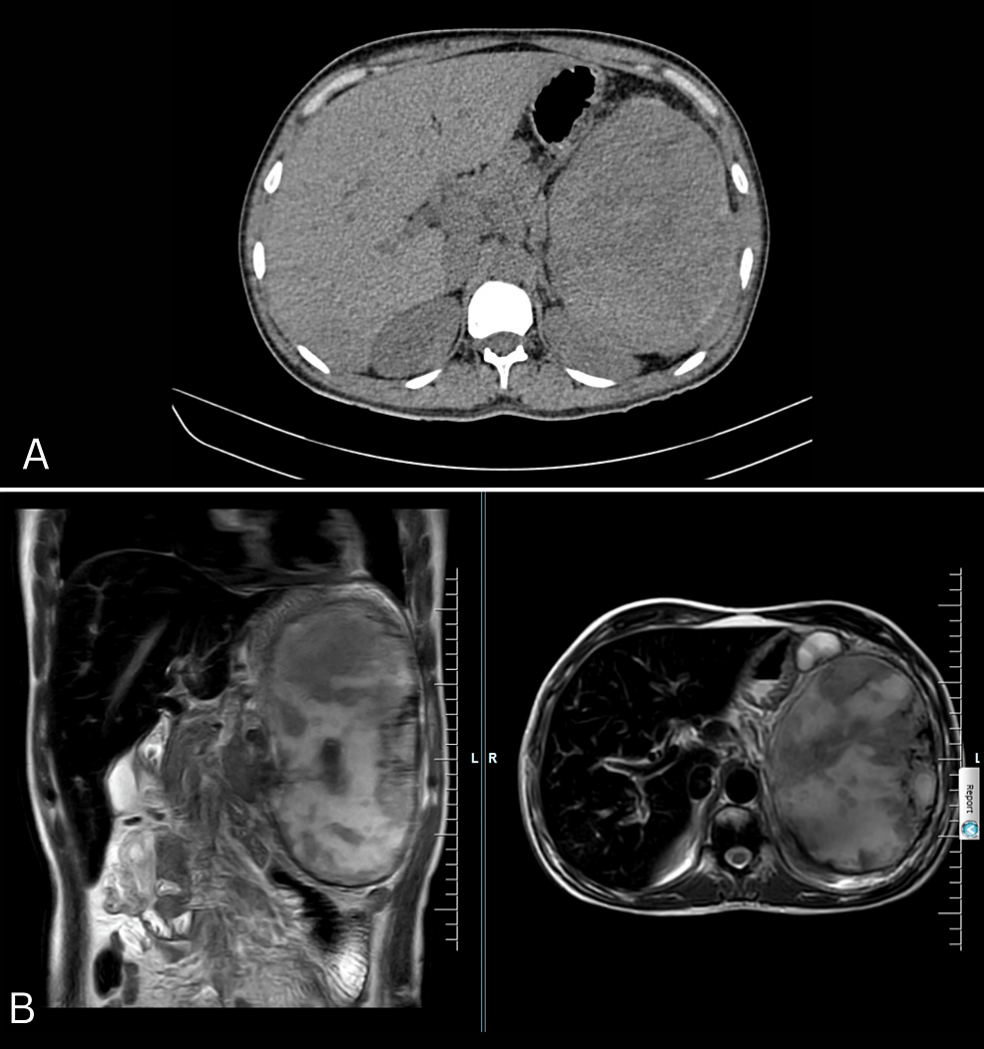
A) CECT abdomen showing hemangioma in the spleen with splenomegaly and hepatomegaly; B) MRCP suggests cholelithiasis, hepatomegaly with focal haemorrhages, splenomegaly with splenic infarcts with haemorrhage CECT - Contrast-enhanced computed tomography, MRCP - Magnetic resonance cholangiopancreatography
